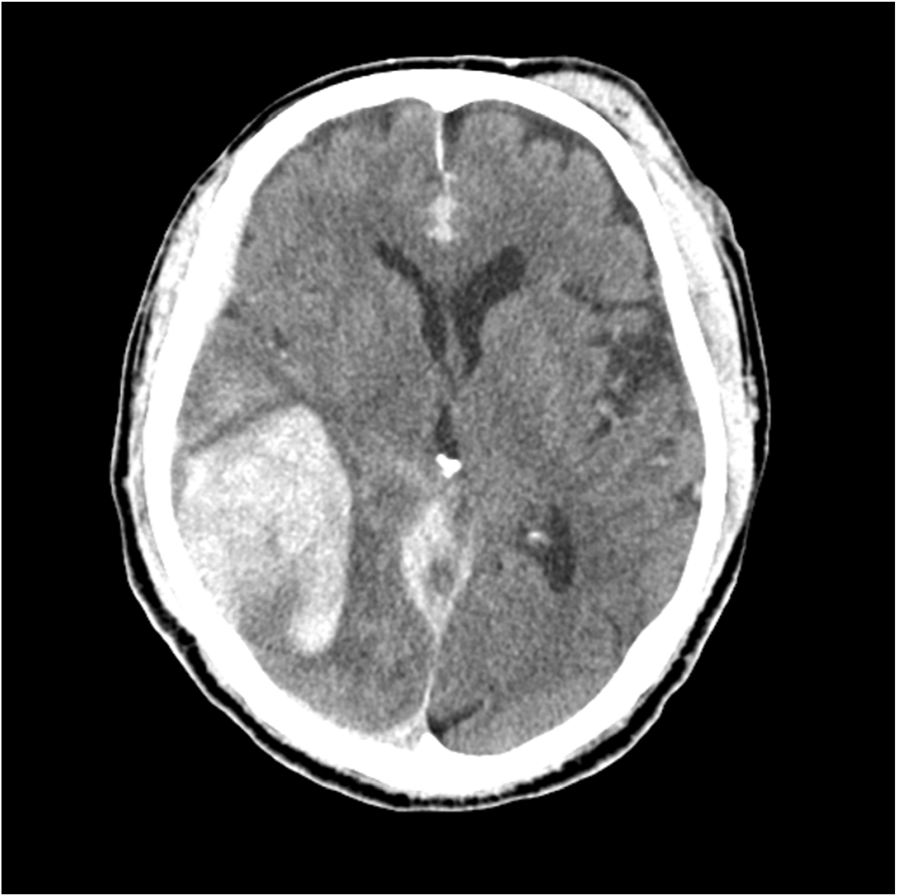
Head CT 3 h after admission showed the expansion of the temporal lobe hematoma
